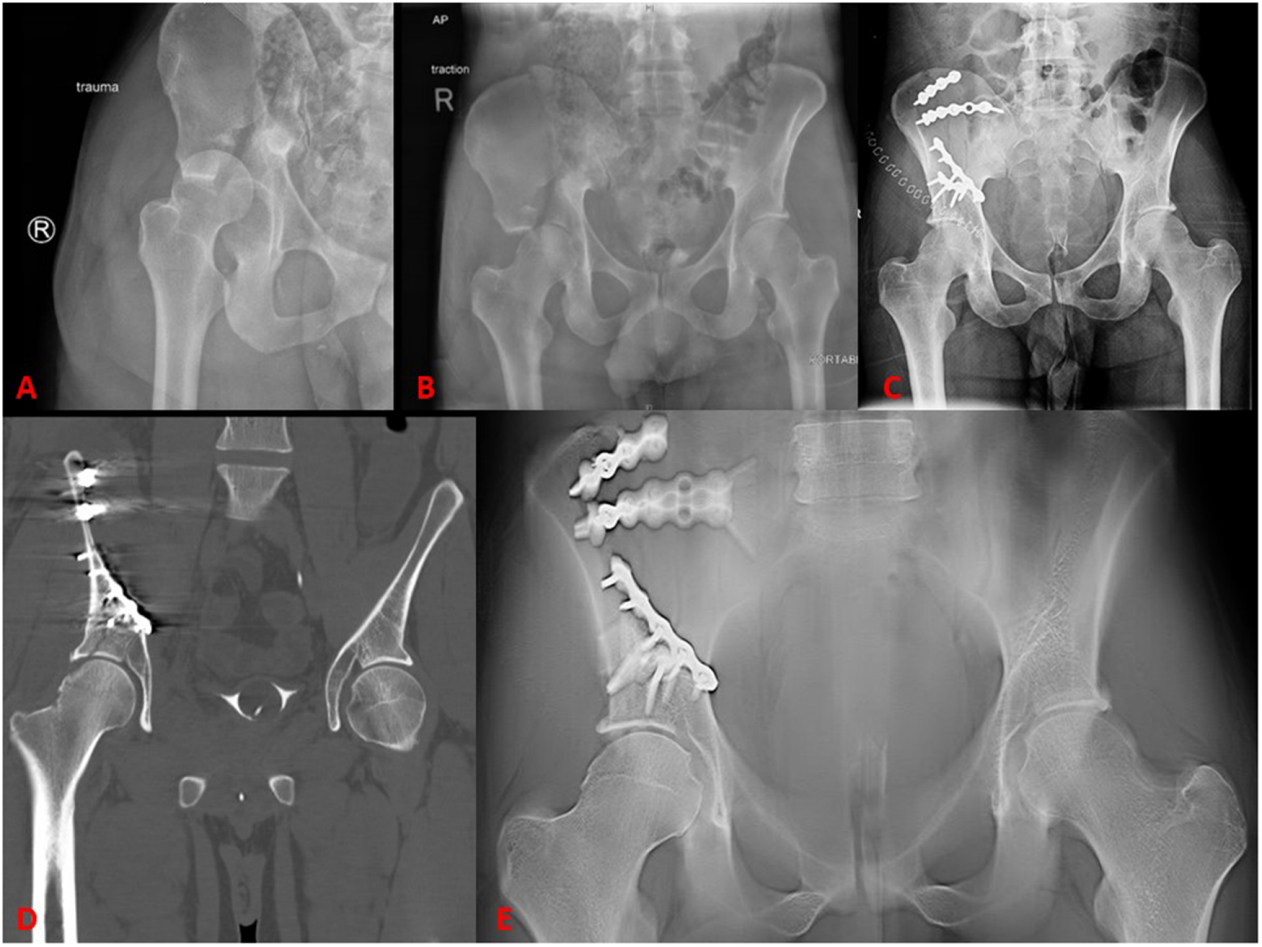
AP digital radiograph of the right right hip of a 28-year-old male (A) with a R acetabular fracture and hip dislocation. AP pelvis digital radiograph of the same patient (B) following closed reduction. Post-operative AP pelvis digital radiograph (C), representative coronal slice from postoperative computed tomography scan (D) representative coronal slice from digital tomogram (E).
